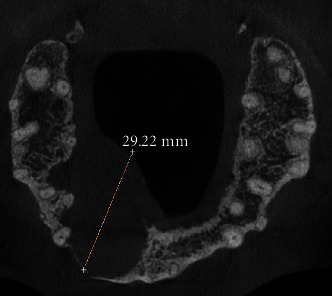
CBCT axial section showing the rupture of the internal and external bony cortices caused by the extension of the periapical lesion.
